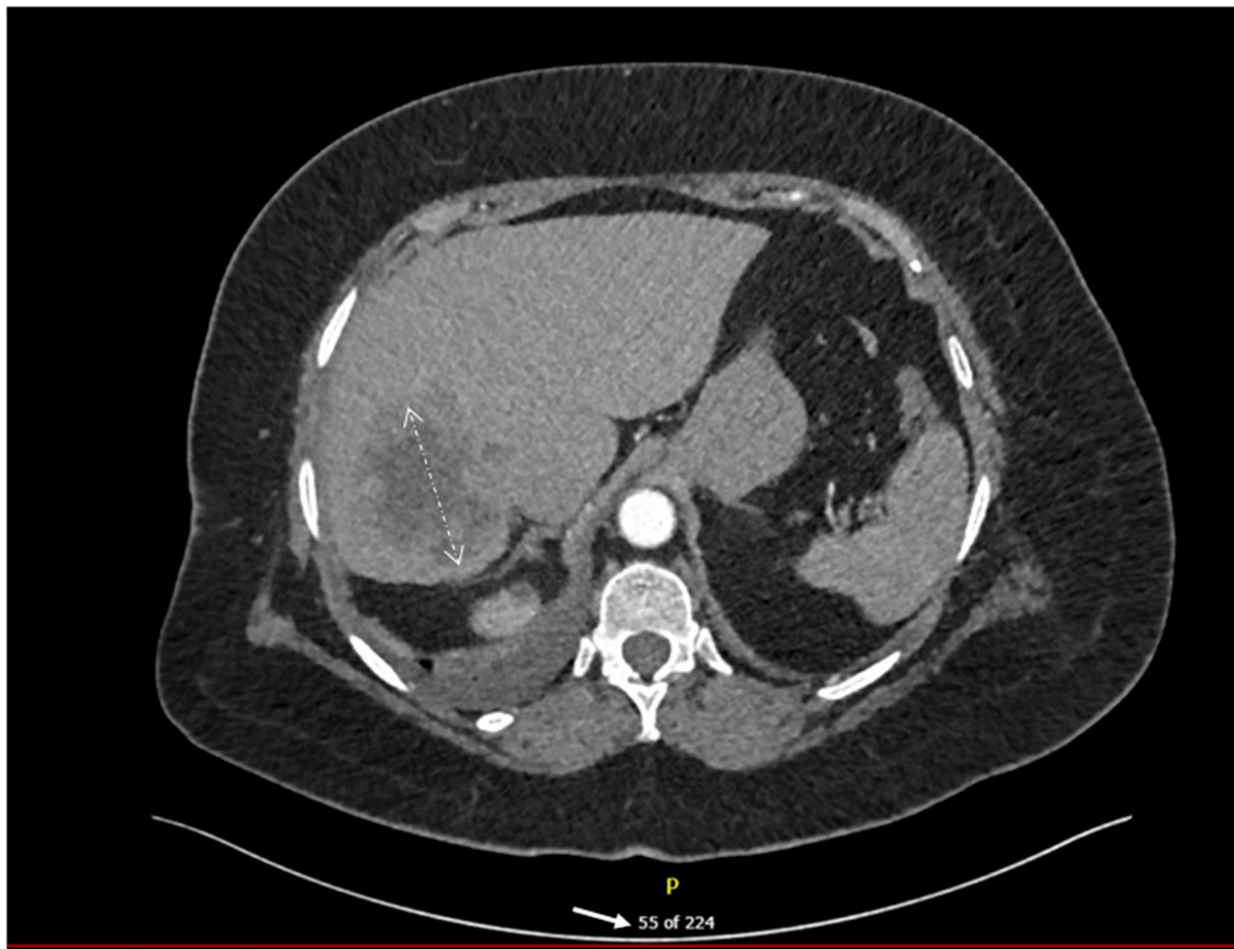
CTPA image showing extent of lesion CTPA: Computed tomography pulmonary angiography Bilateral hepatic cysts, measuring 12 mm on the right, with a left interpolar one at 2 cm.
